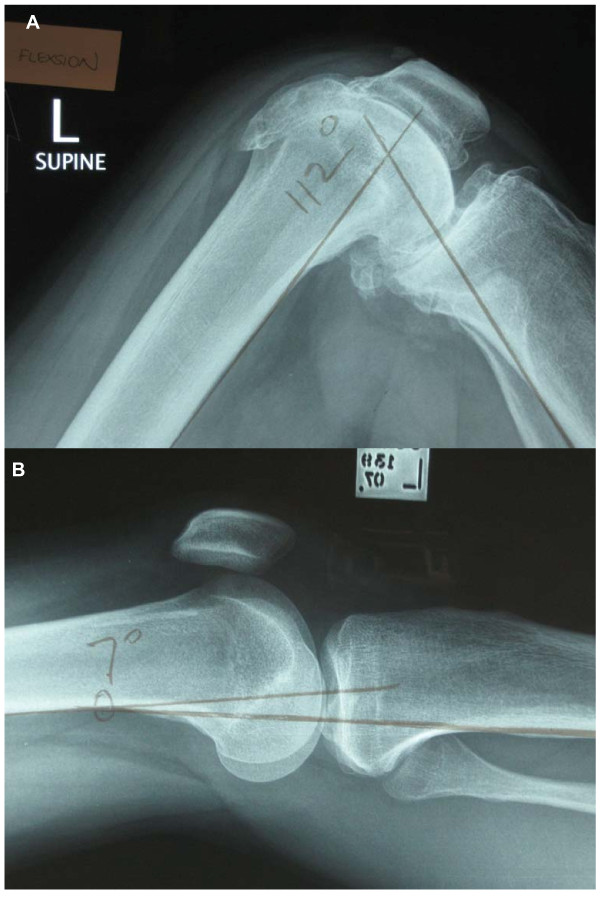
Patient x-rays demonstrating measurement of angles in flexion and extension.
